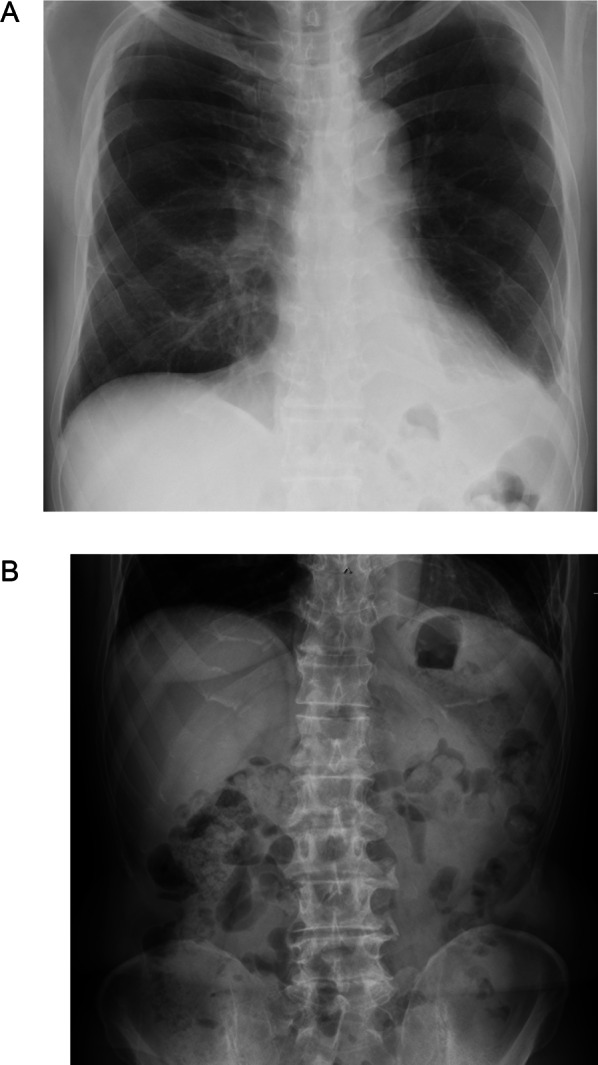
Postoperative radiographs. Chest (A) and abdominal (B) radiographs do not show prolapse of intra-abdominal tissue into thoracic cavity
